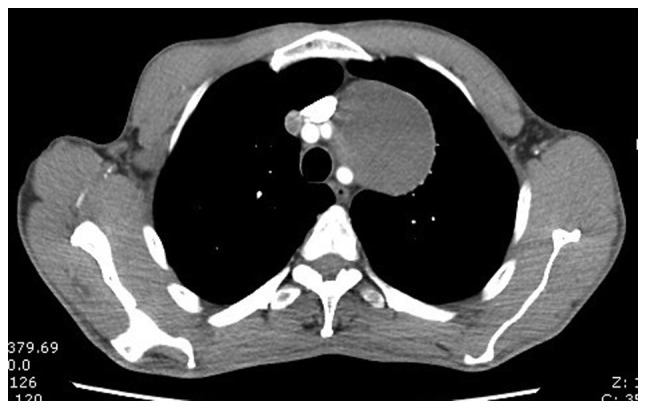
Contrast-enhanced computed tomography of the chest showing the circumscribed mass identifiable in the left superior mediastinum with a smooth and clear margin, and measuring 78×66×59 mm.
